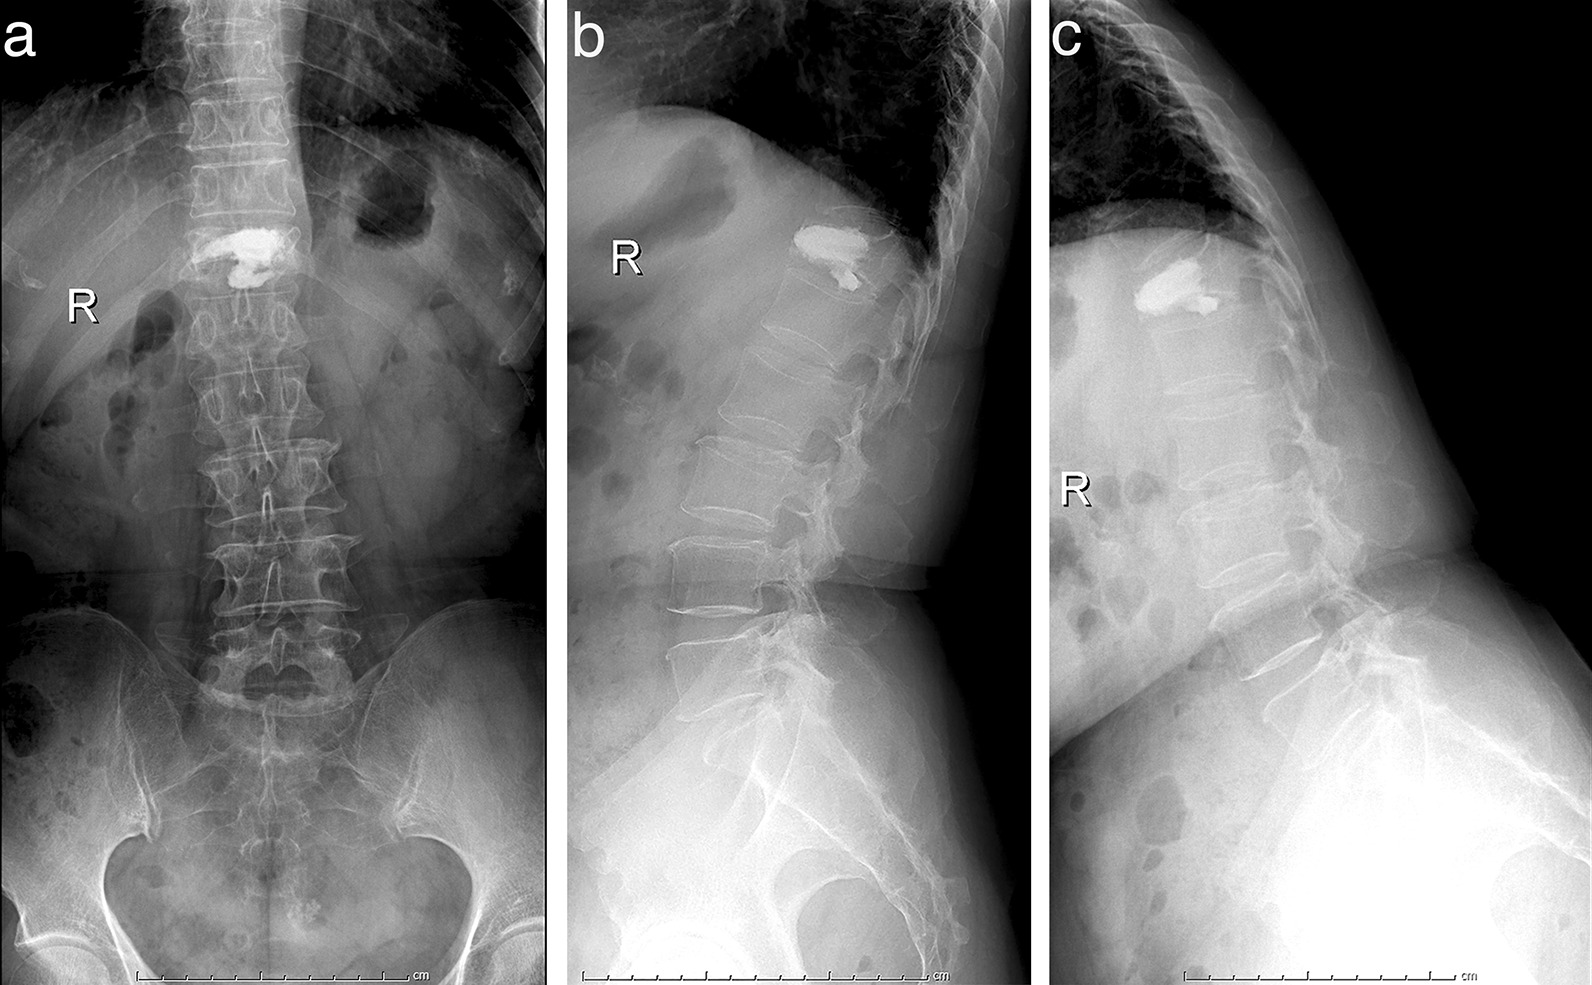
The radiographic data included lumbar anteroposterior (a), lateral (b) and lateral hyperflexion (c) lumbar X-rays
